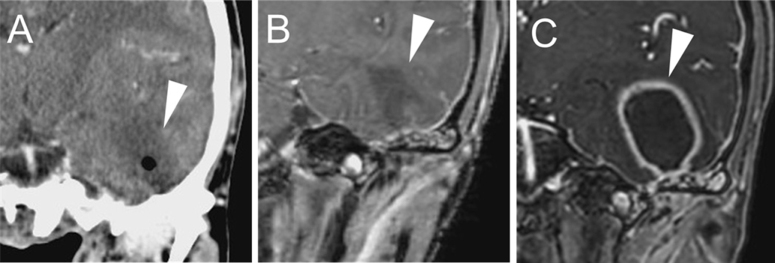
Imaging findings of the left temporal lobe. (A) Follow-up computed tomography (day 1 of hospitalization): Intracranial gas was observed. (B) Follow-up magnetic resonance imaging (MRI; day 1 of hospitalization): A low-intensity area was observed on T2-weighted imaging. (C) Follow-up MRI (day 18 of hospitalization): Abscess formation was observed.
